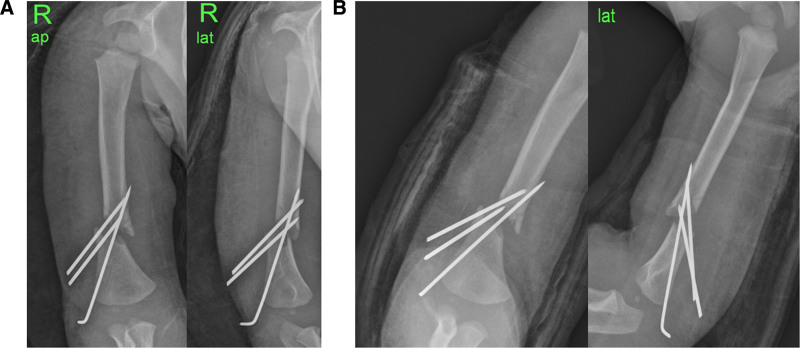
(A) Reduction loss was identified on immediate postoperative radiographs. (B) Increased reduction loss and migration of Steinmann pins were confirmed on radiographs taken the day after the first surgery.
